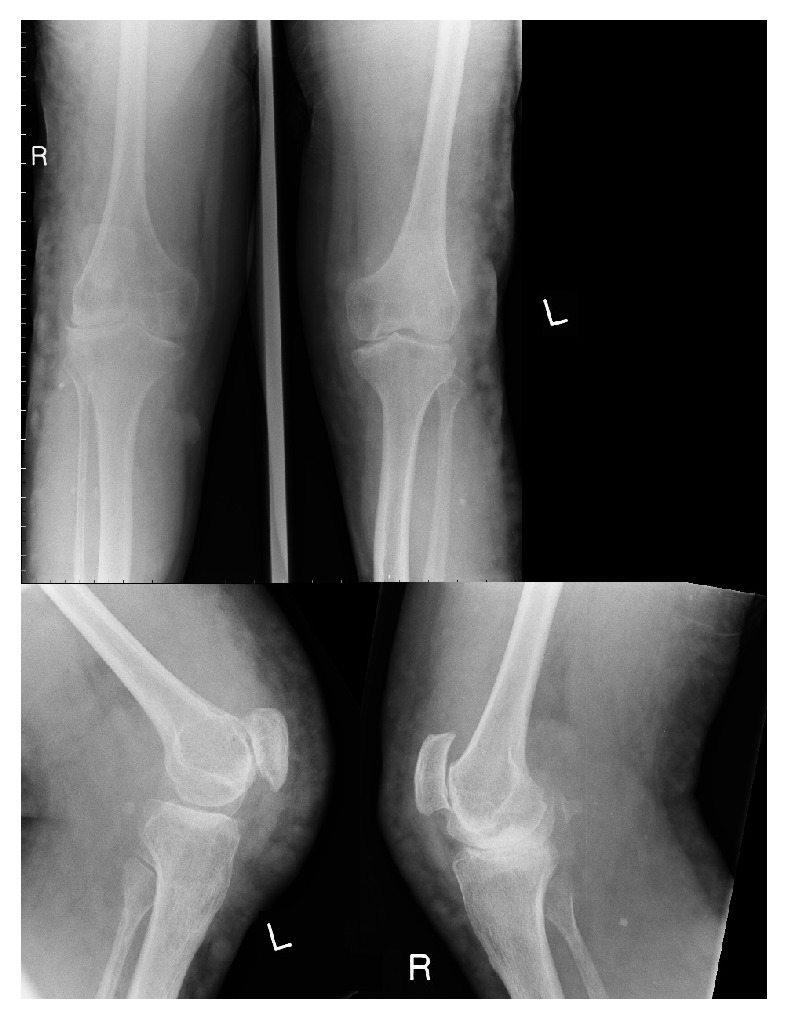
AP and lateral radiographs of the patient's knees at age 21.
